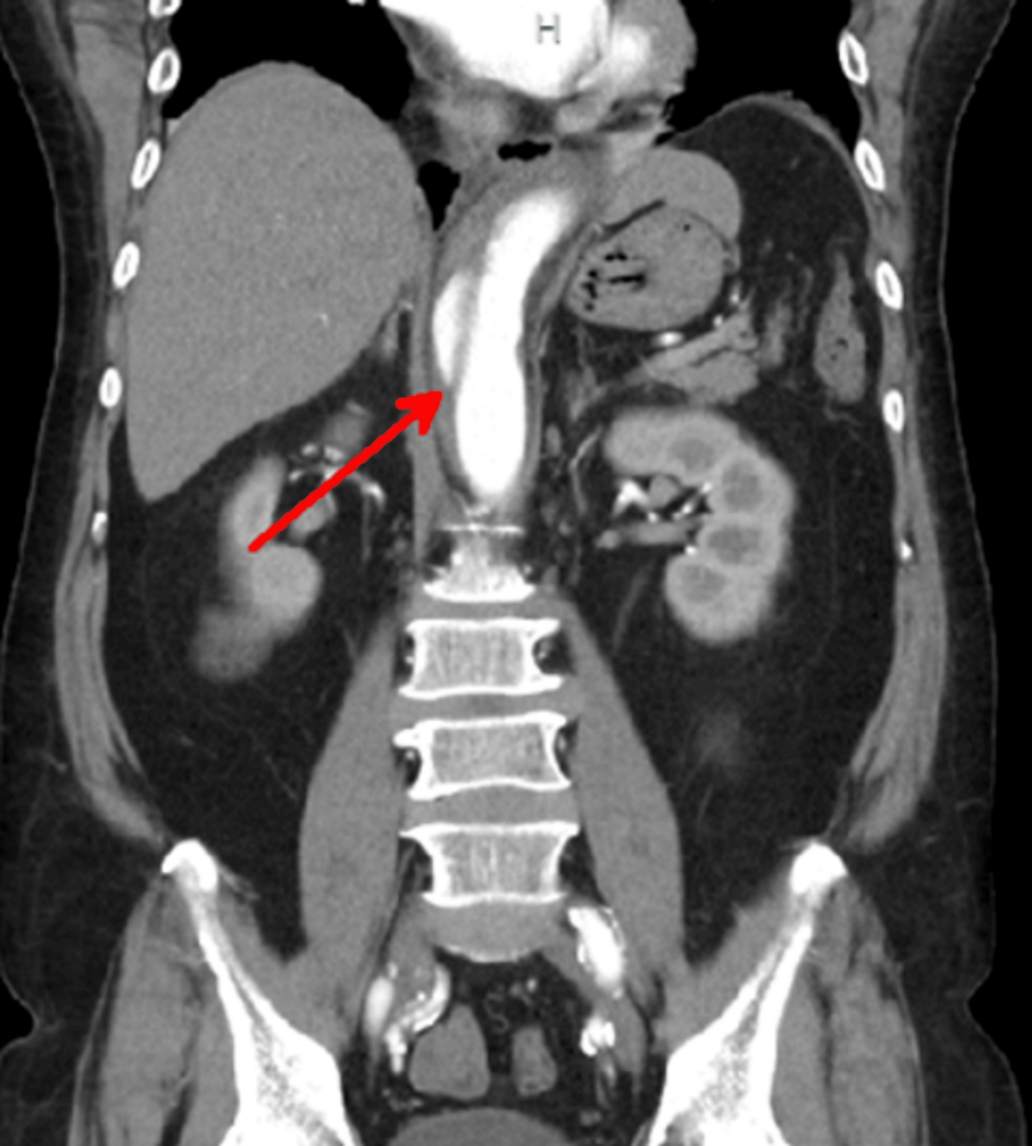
Abdominal computed tomography (CT) scan with contrast enhancement in coronal view The abdominal CT scan reveals a false lumen in the abdominal aorta (red arrow).
